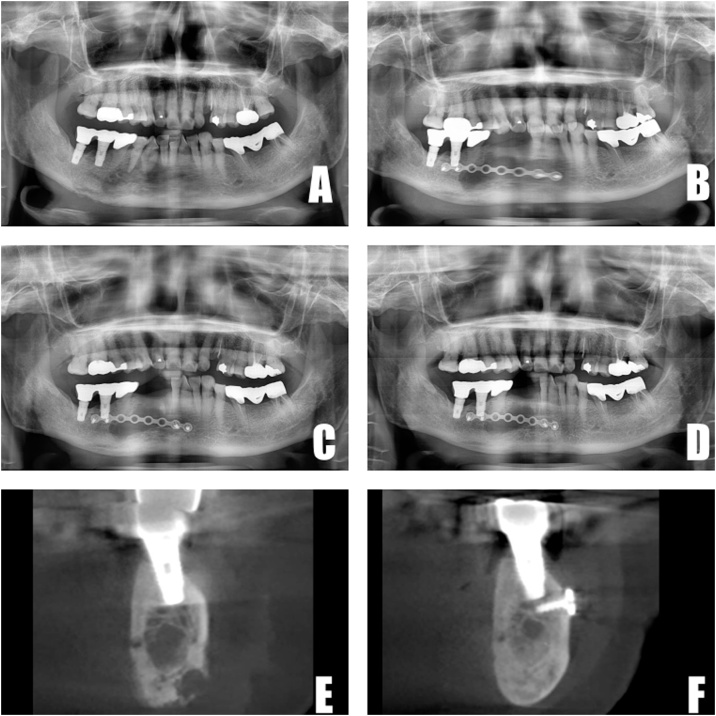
A. Panoramic X-ray at first visit. B. Panoramic X-ray 1 month postoperatively. C. Panoramic X-ray 3 months postoperatively. D. Panoramic X-ray 6 months postoperatively. E. CBCT at first visit. F. CBCT 6 months postoperatively.
